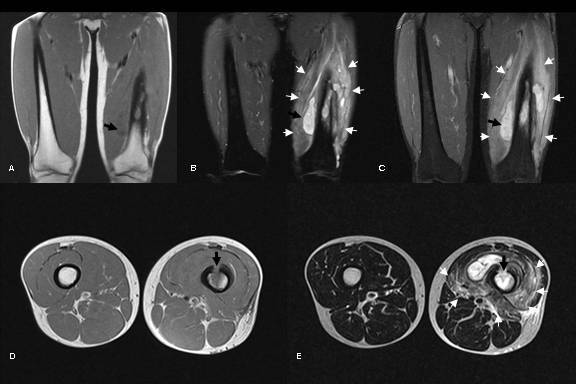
Coronal images showing mass-like lesions (black arrows) along left femoral shaft which were isointense to skeletal muscles on T1-weighted images (A), hyperintense on STIR images (B) and homogenously enhancing post-gadolinium (C). Axial T1-weighted images (D) and T2-weighted images (E) showing thickened anterior cortex with a focal break forming a cloaca (black arrows). Ill-defined areas of high signal changes on T2-weighted, STIR and post-gadolinium images in the marrow cavity at previous RFA site and in the adjacent muscles reflects inflammation and oedema.
